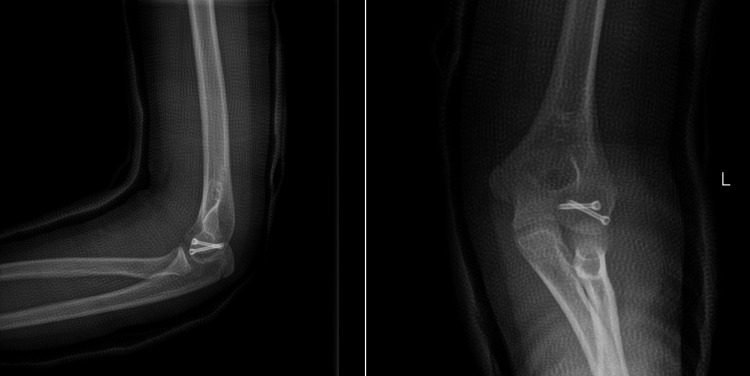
Anteroposterior and lateral radiographs of the open reduction and internal fixation of the capitellar fracture with headless screws were obtained two weeks postoperatively.
